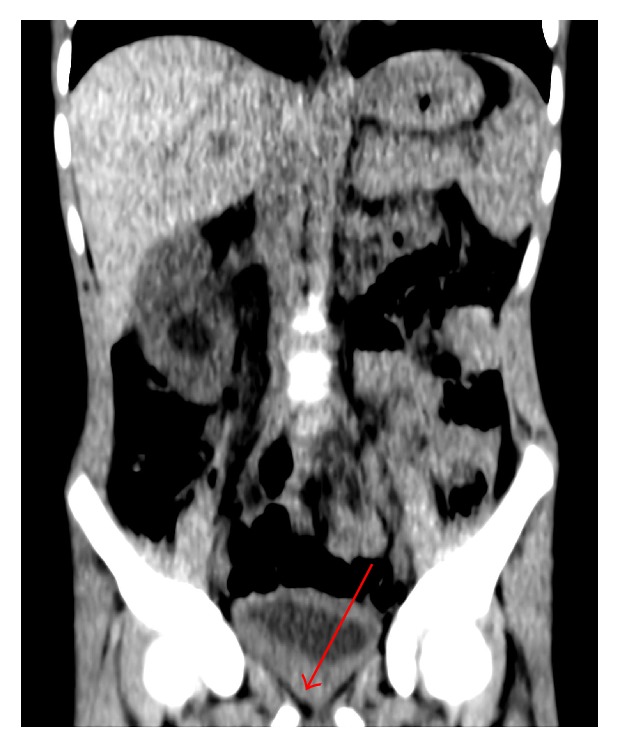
CT confirmed the finding of irregular bladder wall thickening but did not characterize the bladder lesion as well as US. Coronal reconstruction with 8 mm slice thickness and digital optimization.
